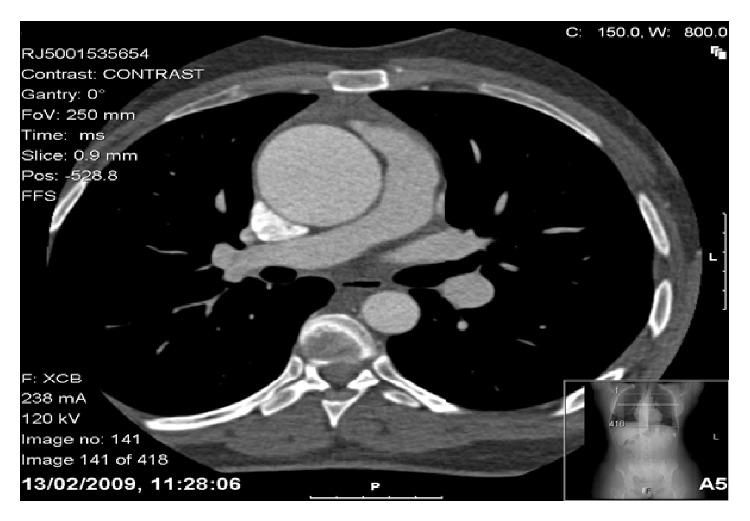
Contrast CT image showing large ascending aortic aneurysm measuring 6.3 × 5.4 cm.
